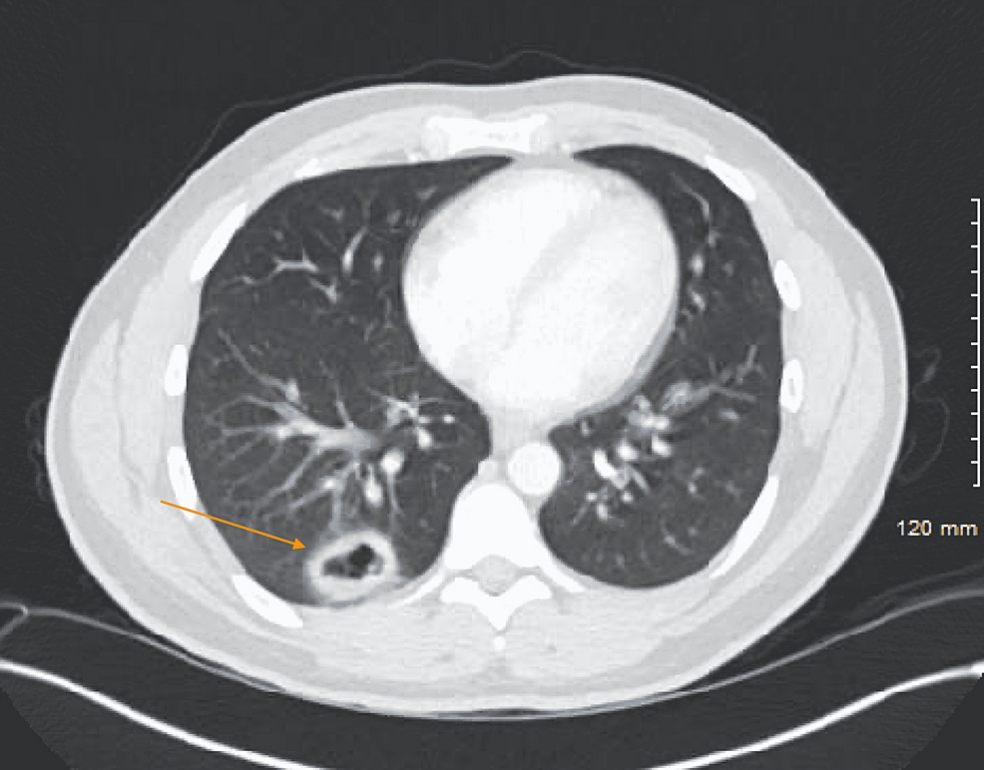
CT scan of the chest revealing the presence of 2.9 x 3.7 cm posterior right lower lobe cavitary nodule (as indicated by the arrow) as well as multiple smaller bilateral pulmonary nodules with shaggy indistinct margins.
